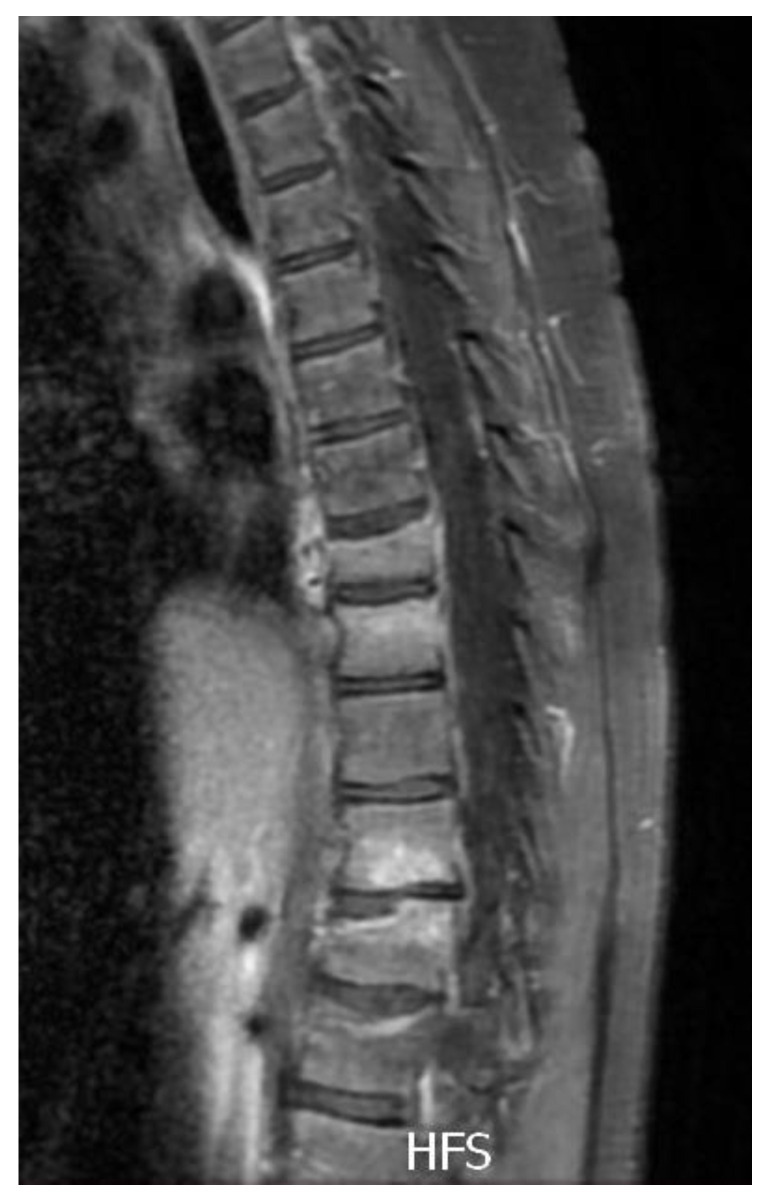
MRI of the spine depicting the vertebral lesions prior to the pamidronate treatment.
